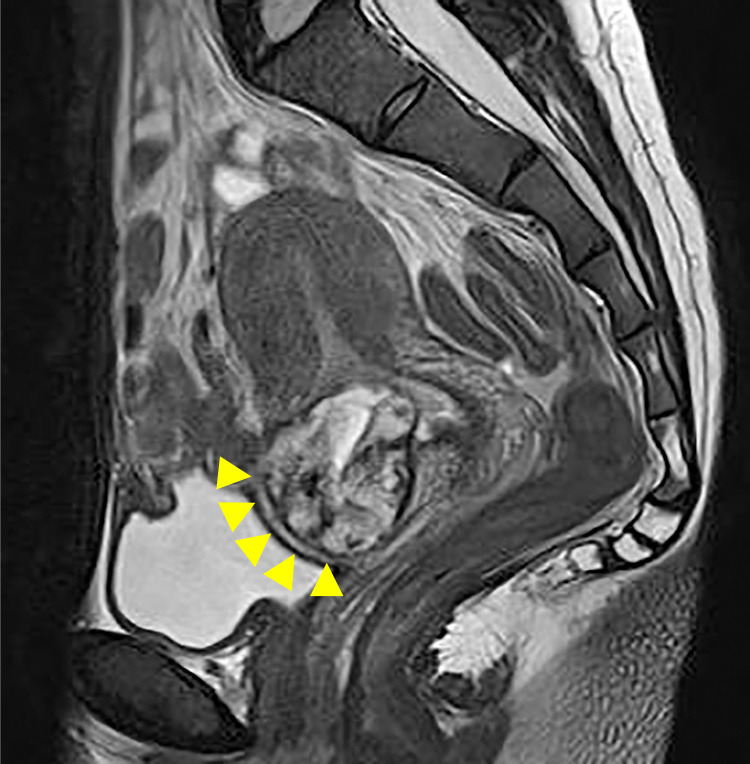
Simple MRI T2-weighted sagittal section: cesarean scar pregnancy (CSP) findings (arrowhead).
